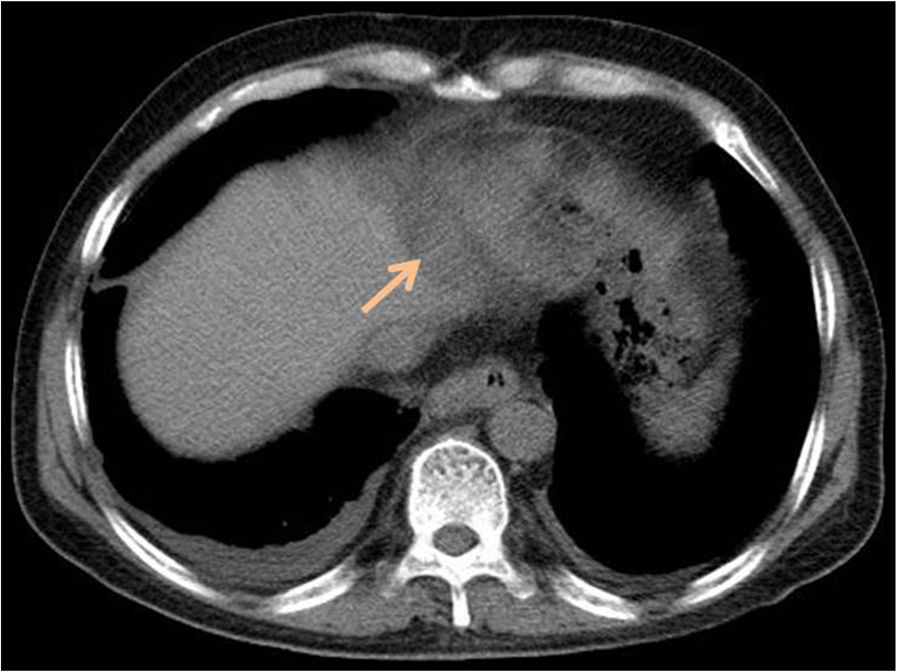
Computed tomography scan images obtained 3 day after cryoablation. The *arrow* indicate the ablated zone
